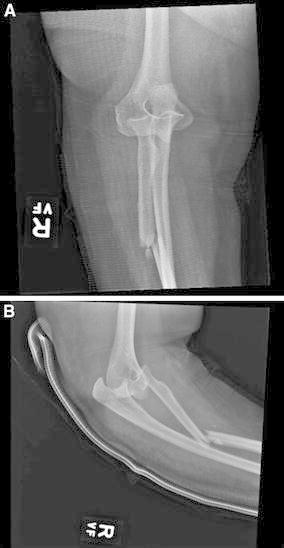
AP (a) and lateral (b) radiographs of the right elbow reveal a fracture of the radial shaft with the apex dorsal. The proximal radius is dislocated anteriorly and the ulna posteriorly with respect to the humerus
